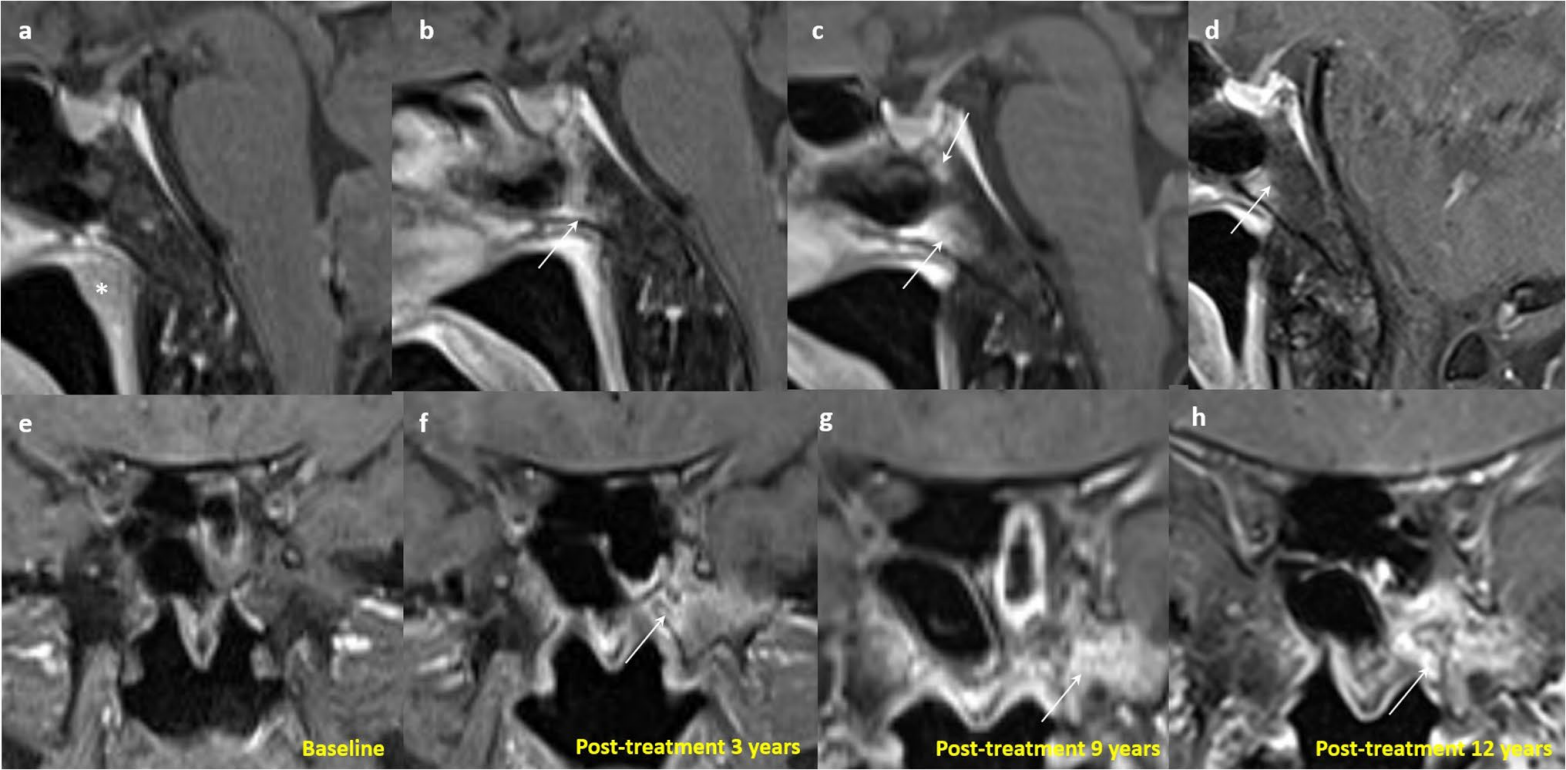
Serial MRI demonstrating the signal change in the basisphenoid of a 56-year-old female with a history of localized NPC (clinical stage T1N0M0) in 2011 with pathological complete remission. Sagittal T1-weighted post-contrast MRI with fat suppression at baseline shows no evidence of signal abnormality at the clivus. The nasopharyngeal tumor is seen (asterisk) (a). There is progressive increase in the patchy enhancement in the clivus in 3-year and 9-year follow–up images (b) and (c), and the signal abnormality shows partial resolution in 12-year follow-up image (arrows) (d). Coronal T1 post-contrast MRI with fat suppression at baseline shows no evidence of signal abnormality at the basisphenoid (e). There is progressive increase in the patchy enhancement in the bilateral basisphenoid, more on left side, in 3-year follow-up image (f) and 9-year follow–up image (g), and the signal abnormality shows partial resolution in 12-year follow-up image (arrows) (h)
